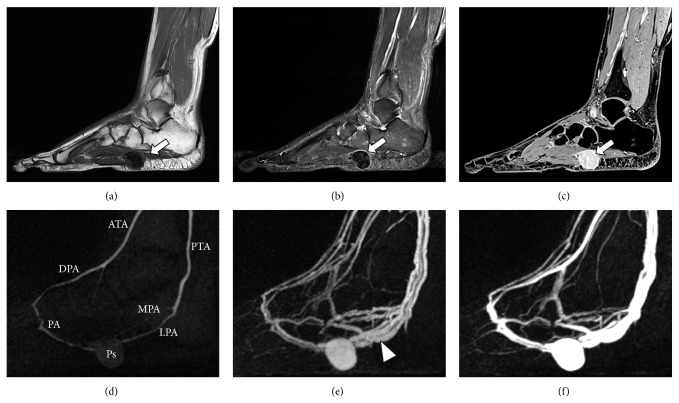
MRI and MRA two months after the trauma (3T Magnetom Skyra, Siemens AG Healthcare, Erlangen, Germany). (a) T1 image. (b) STIR (short-Ti inversion-recovery) image. (c) Gadolinium-enhanced VIBE (volumetric interpolated breath-hold examination) image using 6 mL Gadovist (Bayer Schering, Berlin, Germany). (d)–(f) TWIST (time-resolved angiography with interleaved stochastic trajectories) 3D MRA with a temporal resolution of 5 seconds. White arrow = pseudoaneurysm. White arrowhead = early filling of the veins due to arteriovenous fistula. ATA = anterior tibial artery. DPA = dorsalis pedis artery. PA = plantar arch. MPA = medial plantar artery. LPA = lateral plantar artery. PTA = posterior tibial artery. Ps = pseudoaneurysm of the lateral plantar artery.
